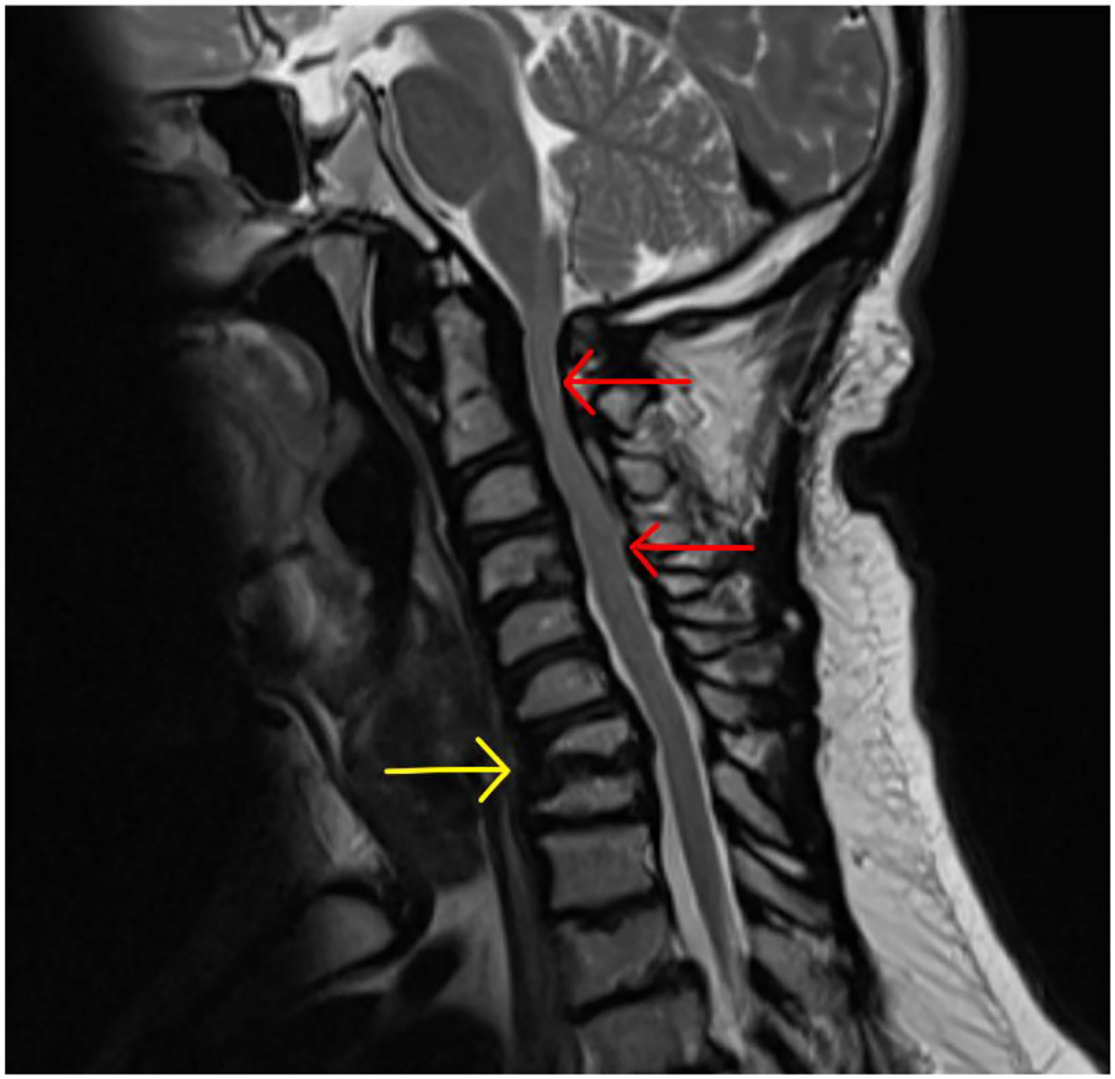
Cervical cord stenosis. Sagittal MRI of adult with MPS I demonstrating cervical cord stenosis at C2–C4 and hypertrophied ligament, with effacement of anterior and posterior CSF spaces (red arrows). Also note the abnormal vertebrae at C7 and T1 with degenerative disc and hypertrophied ligament (yellow arrow) causing cervical-thoracic kyphosis and effacement of anterior CSF spaces.
